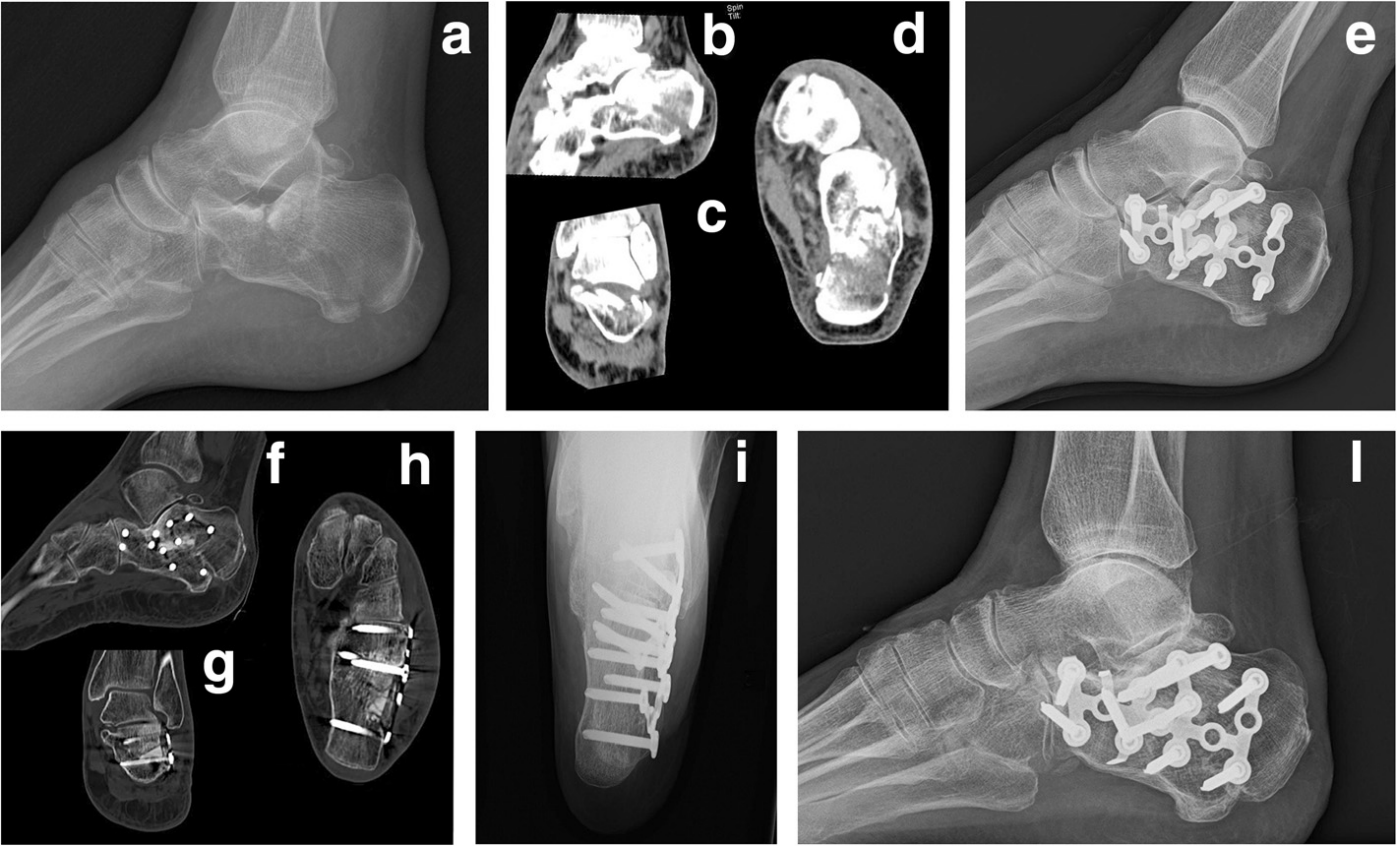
Female 56 years old: (a–d) preoperative X-ray and CT; (e) postoperative check up; (f–h) follow-up 10 months later; (i–l) follow-up 2.5 years later
